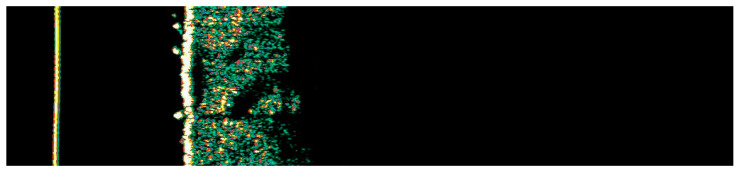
Ultrasonographic image of BCC on the thigh.
